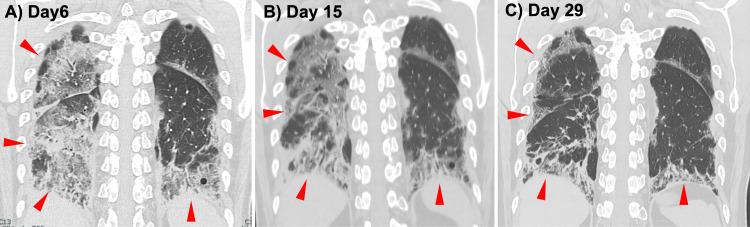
Serial coronal chest CT images over the clinical course (A) Day 6: Extensive bilateral subpleural ground-glass opacities and consolidation, most pronounced in the right lower lobe (arrowheads). (B) Day 15: Decreased ground-glass attenuation with persistent reticulation and early traction bronchiectasis (arrowheads). (C) Day 29: Near resolution of ground-glass changes with established fibrotic reticulation and volume loss in the right lower lobe (arrowheads).
